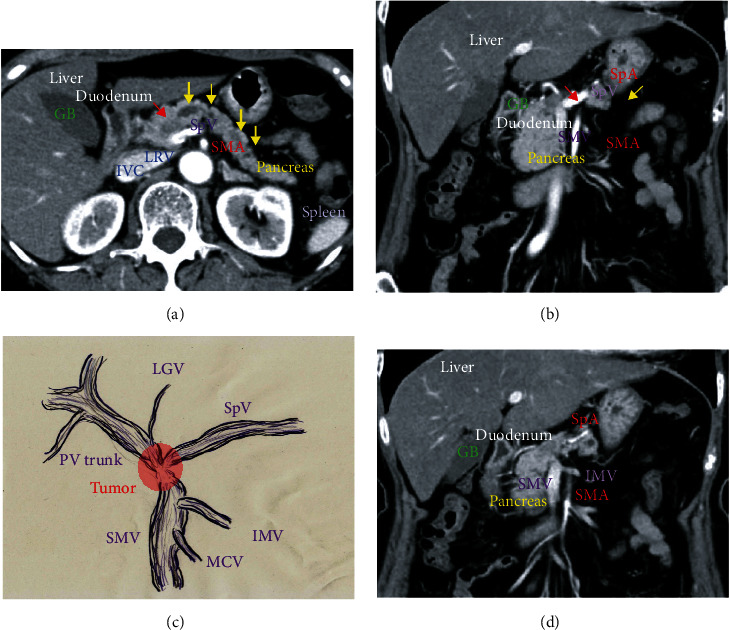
Pancreatic cancer with regional invasion into vessels. (a, b) Enhanced computed tomography shows a hypovascular mass in the pancreatic head (red arrow) and marked dilation of the main pancreatic duct of the distal pancreatic parenchyma (yellow arrows). (c) The pancreatic tumor (red area) markedly involves the confluence of the superior mesenteric vein, portal venous trunk, and splenic vein. (d) The inferior mesenteric vein flows into the superior mesenteric vein, not the splenic vein. There is no evidence of cancer at unresectable sites or distant metastases. GB: gallbladder; IMV: inferior mesenteric vein; LGV: left gastric vein; LRV: left renal vein; IVC: inferior vena cava; MCV: middle colic vein; SMA: superior mesenteric artery; SMV: superior mesenteric vein; SpA: splenic artery; SpV: splenic vein.
